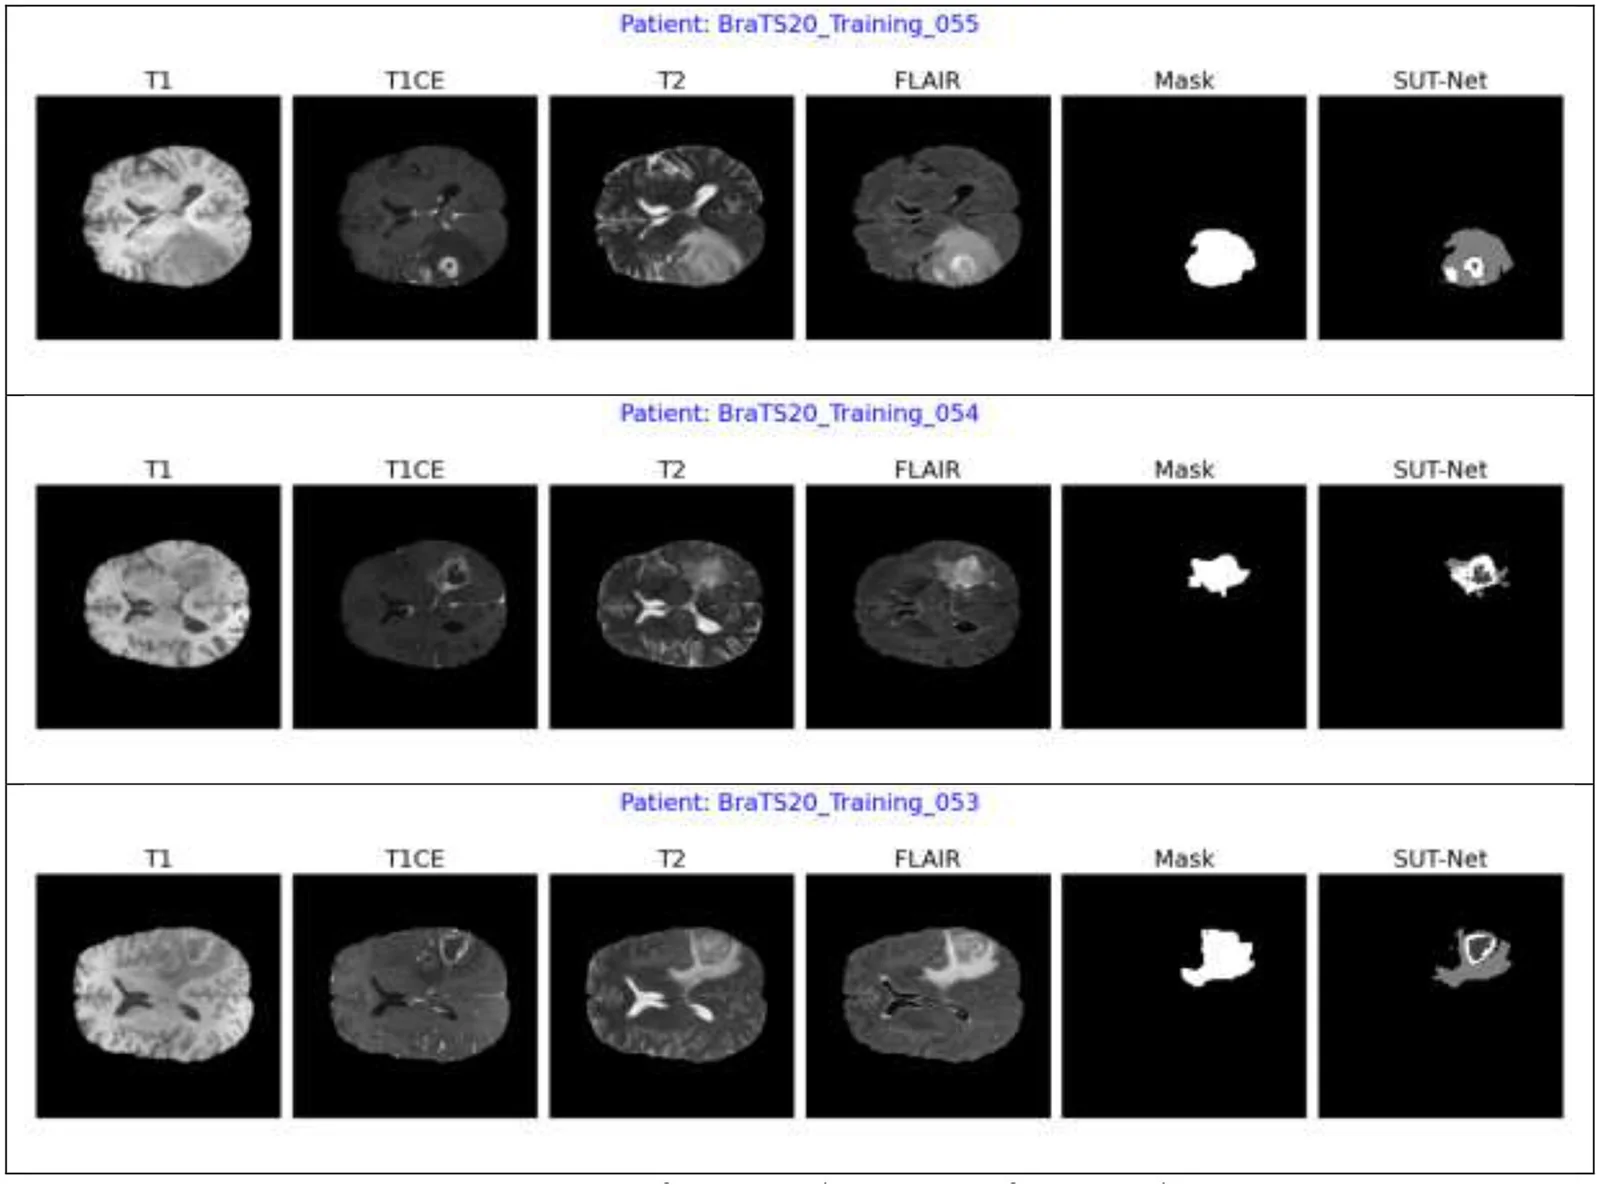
Output of proposed SUT-Net for MRI dataset.
